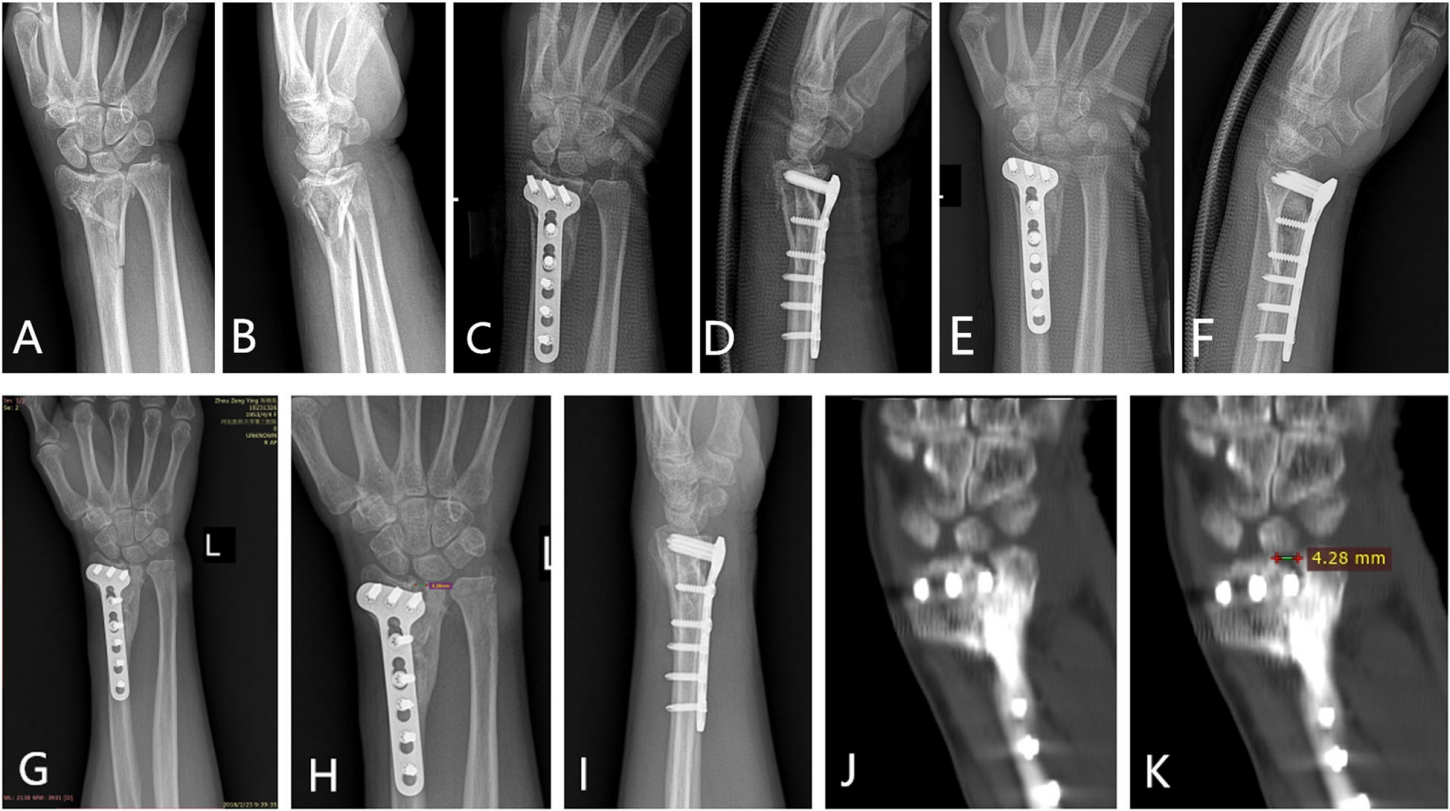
A 57-year woman had her left wrist fractured in an electric bicycle collision injury. The preoperative X-ray (a and b) showed AO type C2 fracture of distal radius. The immediate postoperative X-rays (c and d) showed the acceptable reduction and fixation. Postoperative 1-month X-rays (e and f) showed no evidence of loss of reduction. The postoperative 6-month X-rays and CT scanning showed obvious articular surface gap formation (4.28 mm)
